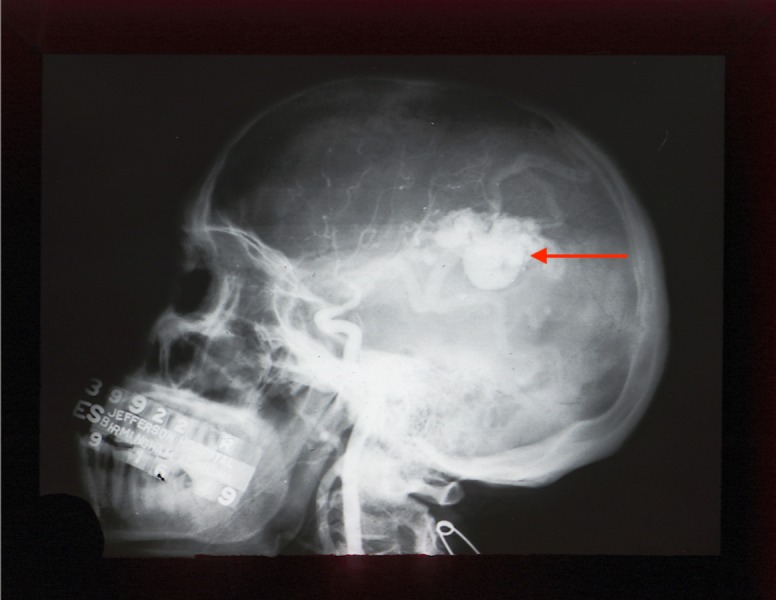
Lateral skull radiograph noting a VGAM (red arrow) VGAM - vein of Galen aneurysmal malformation
